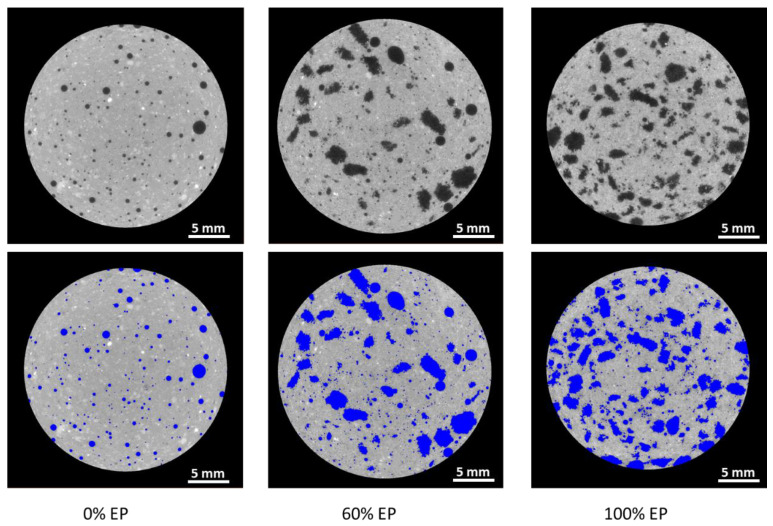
Cross-sectional CT image of block 7. (Top) Raw CT image scan. (Bottom) Thresholding applied to facilitate discrimination between paste particles and air voids/EP particles.
